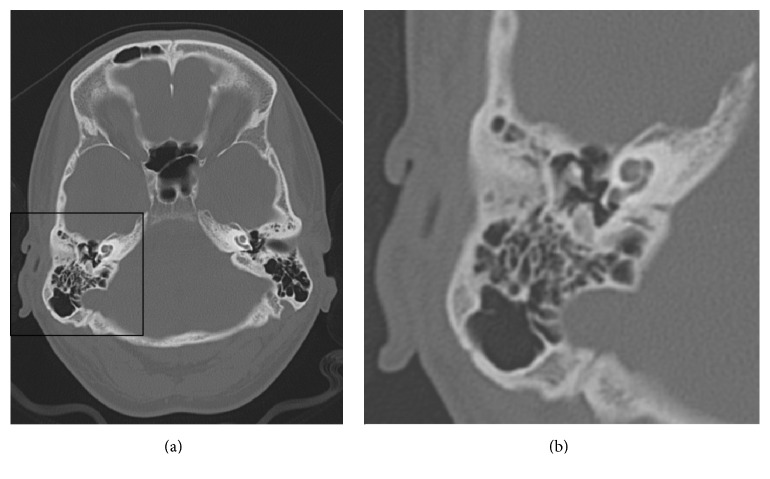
Computed tomography (CT) scan (axial section): No radiological evidence of temporal bone fracture, intact ossicular chains, no hemorrhages, and no obvious fistulas.
